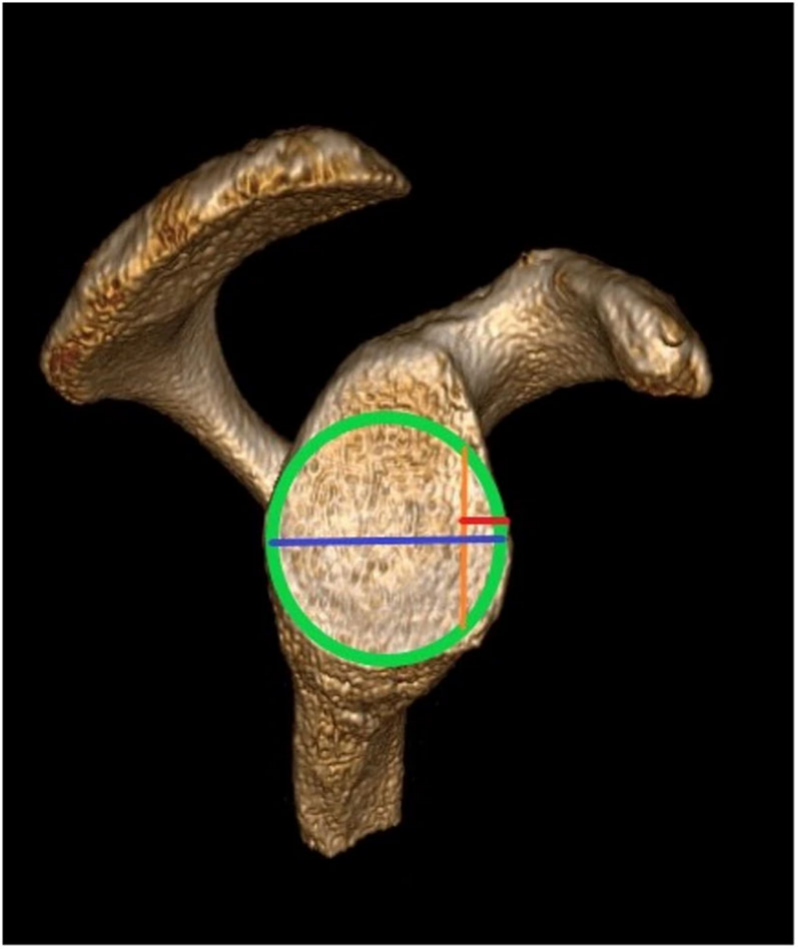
Preoperative CT image in 3D. Diameter of best‐fit circle (green), glenoid width (blue), 20% calculated glenoid bone defect (red/orange). Ref mangler.
